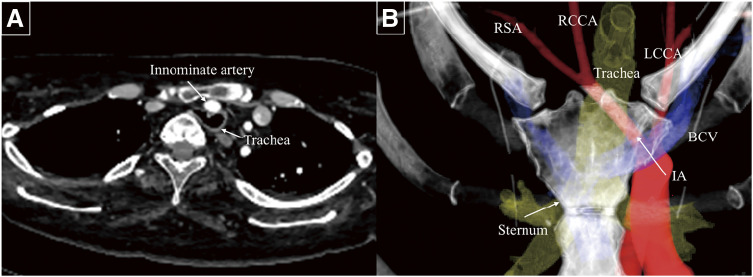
Preoperative contrast-enhanced computed tomography. (A) A 10-mm space between the sternum and vertebral bodies with tracheal compression from the adjacent innominate artery. (B) Three-dimensional computed tomography showing the tracheostomy site, sternum, and major arteries; the aortic arch and innominate artery origin were displaced leftward. LCCA: left common carotid artery; BCV: brachiocephalic vein; RCCA: right common carotid artery; RSA: right subclavian artery; IA: innominate artery
